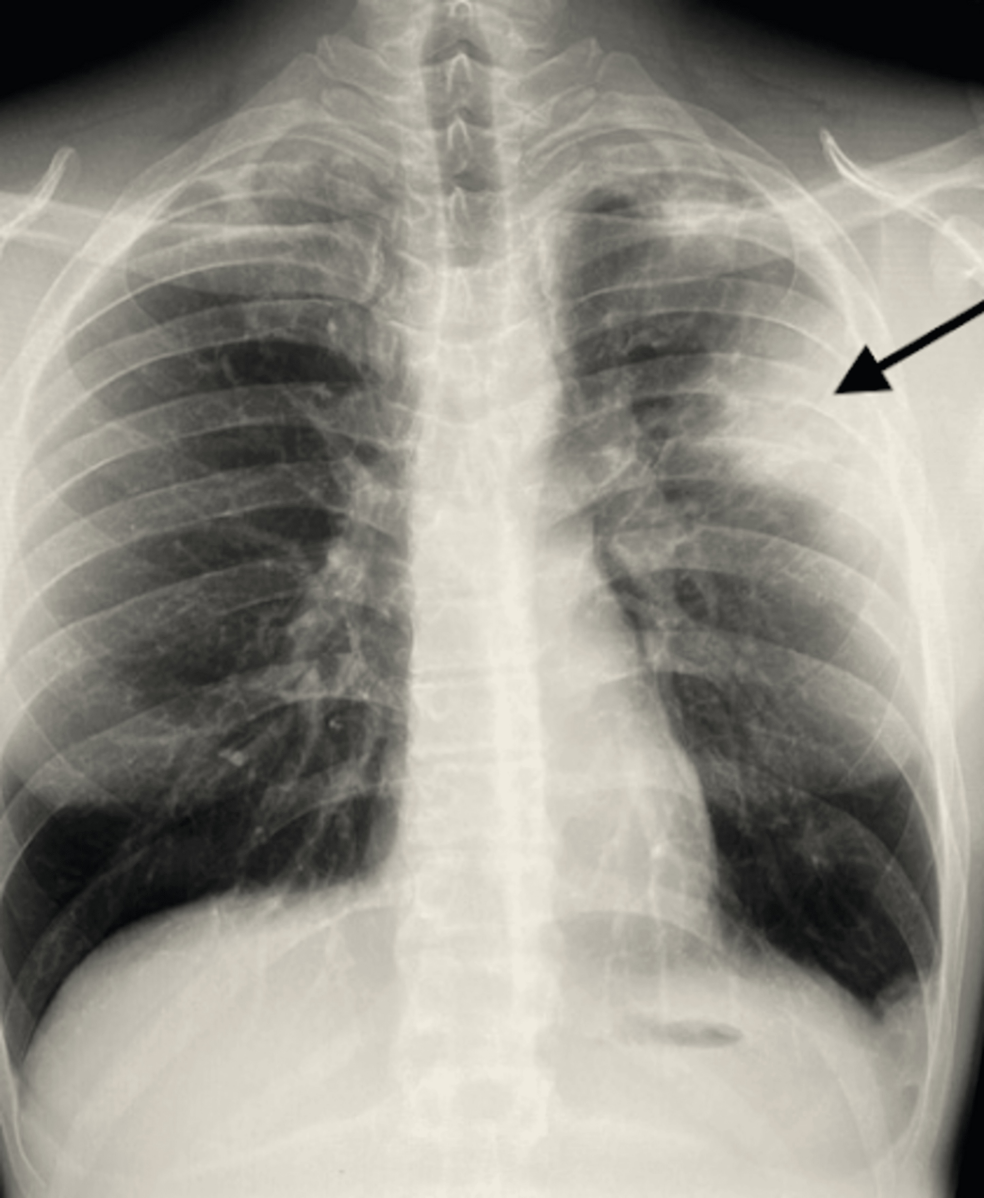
Chest X-ray done on 5/16/2022 showing a unilateral homogenous non-segmental left upper lobe Loeffler-type consolidation pattern (black arrow).
